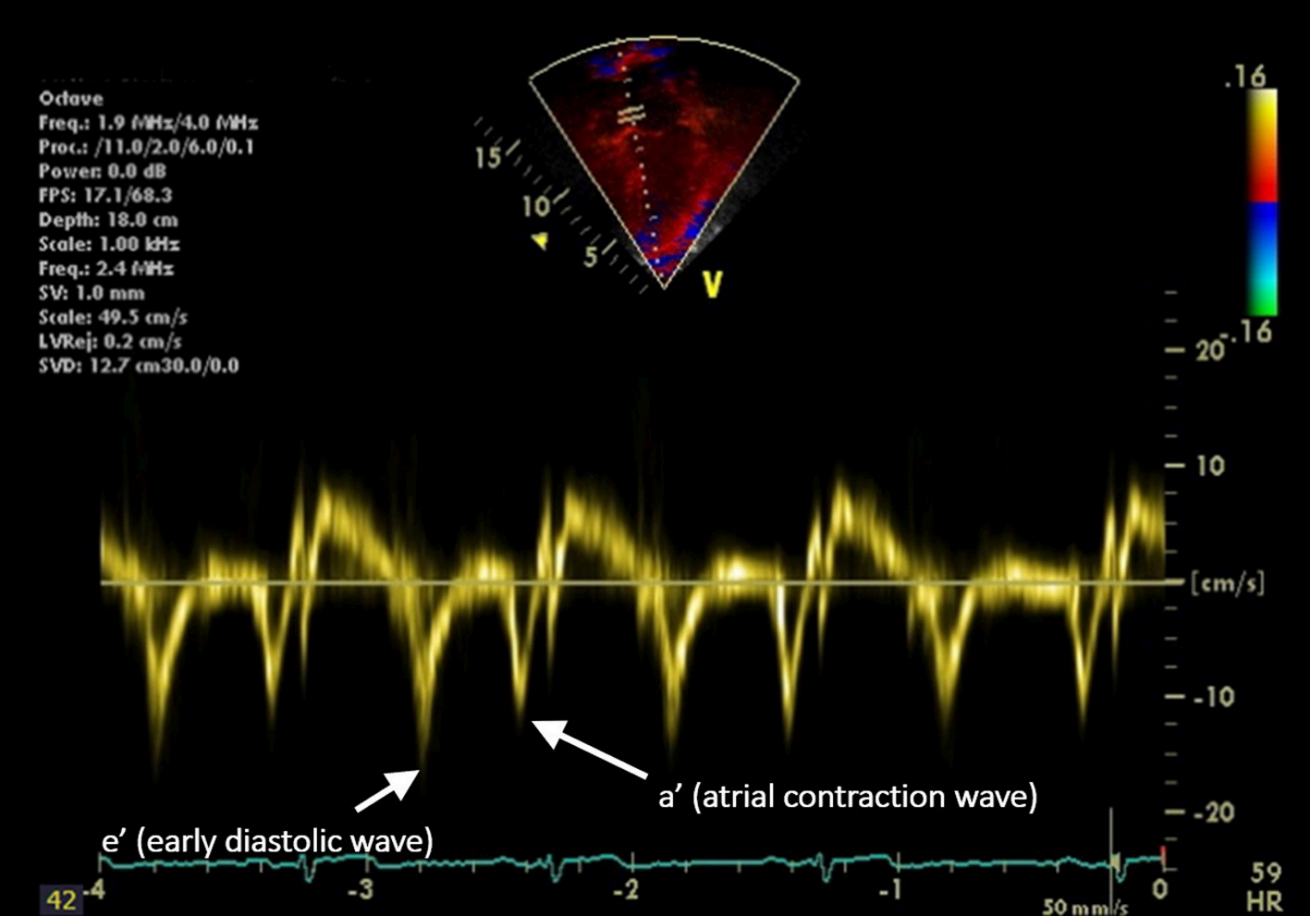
Longitudinal tissue Doppler imaging (TDI) obtained from an apical four-chamber view. The septal portion of the mitral valve is sampled. The peak early diastolic velocity (e′) and the peak late diastolic velocity (a′, representing atrial contraction) are demonstrated.
